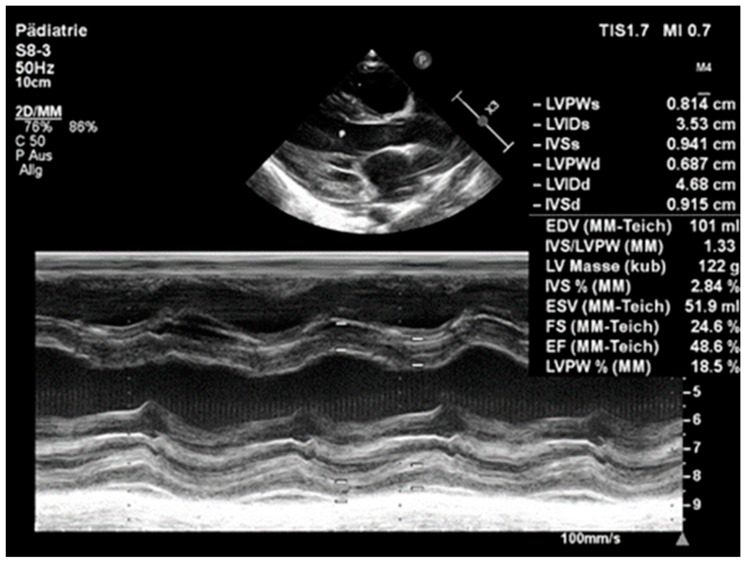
Echocardiography on admission: left ventricle is significantly enlarged (LV enddiastolic diameter of 4.68 (Z-score +4.7) and reduced systolic function (EF 48.6%).
